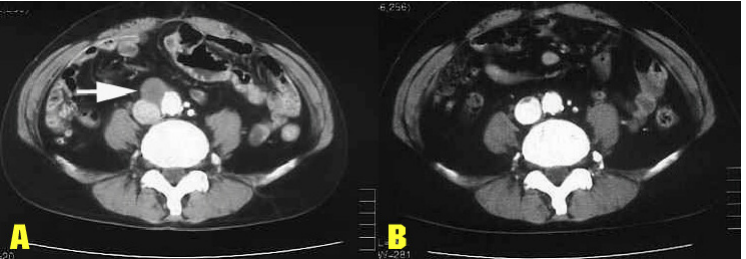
Computerised tomographic scan before and after treatment. A) Abdominal CT showed multiple lymph node swelling, suggesting the presence of metastases (arrowhead). B) Abdominal CT showed the lymph nodes metastases to have completely disappeared.
